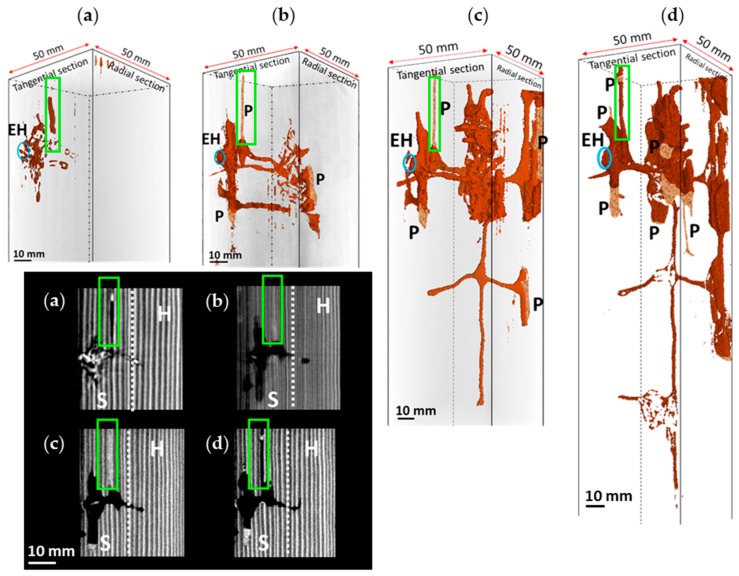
The 3D and 2D CT images of nest-gallery development of Spruce A timber: (a) initial scan; (b) half-year later; (c) one year later; and (d) one and a half years later. Two-dimensional radial-section images show dynamic change of the nest-gallery system, indicated by the sealing and re-opening of a tunnel gallery (green rectangles). In 3D images, the presence of termites is indicated by the uncolored area inside the nest-gallery. In the 2D CT images, the gray value of a pixel corresponds to an index of density: the lighter the color, the denser the area [11]. Springwood and summerwood are indicated by the darker and lighter colors in the images, respectively. EH, entrance hole; S, sapwood; H, heartwood; P, fecal pellets.
